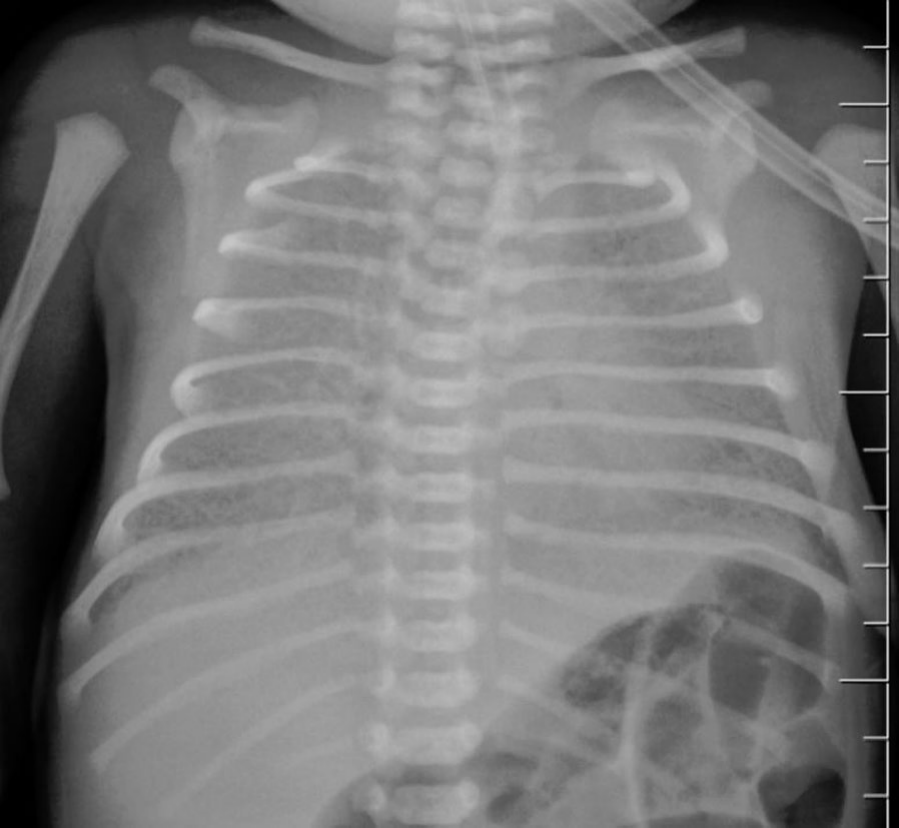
Chest X-ray, front view. Total infiltration of both lungs is determined mainly due to the compaction of the pulmonary interstitium
